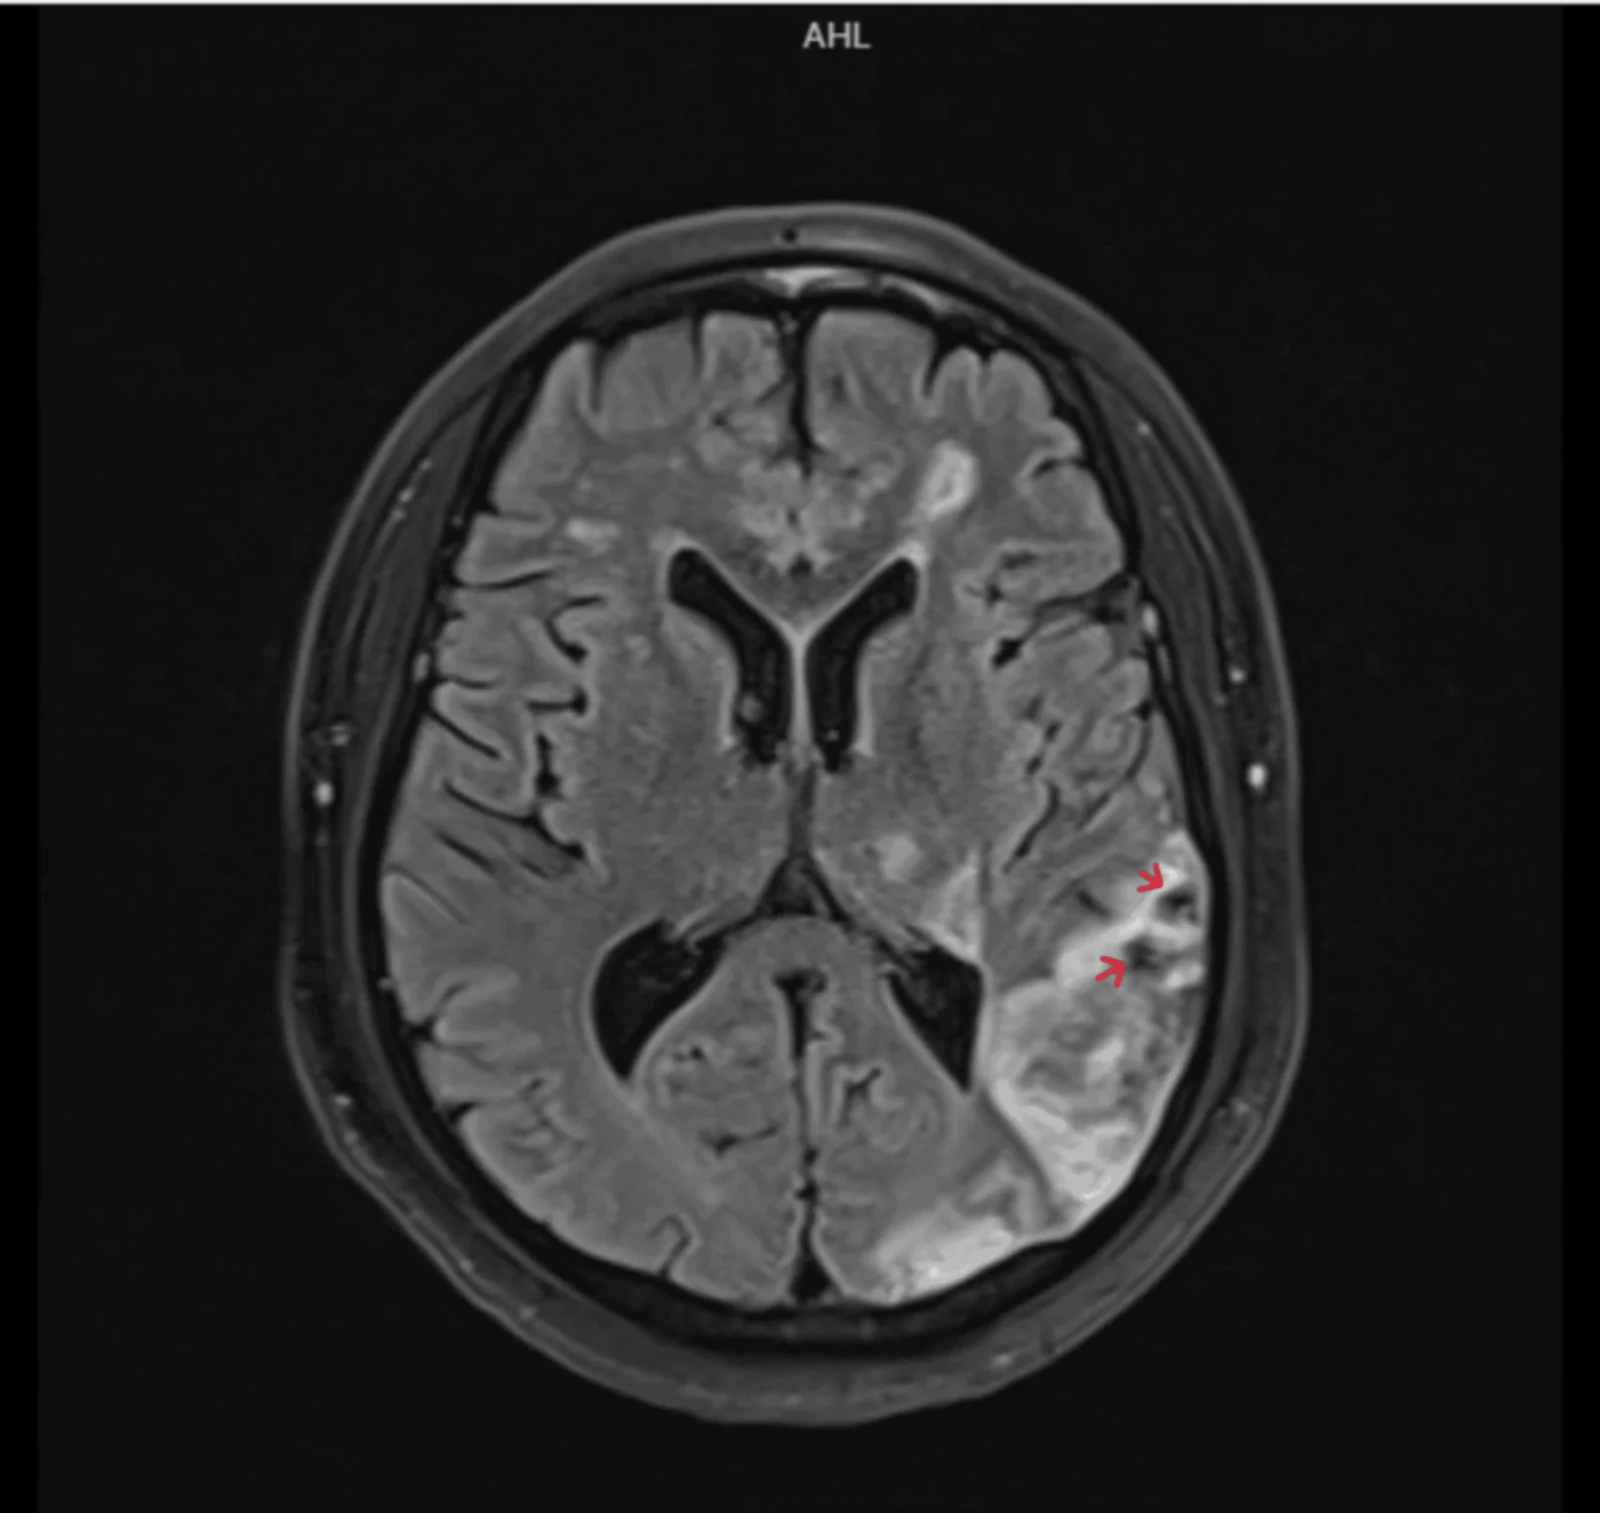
Post-thrombectomy MRI demonstrating acute left ICA territory infarction involving the hemisphere and thalamus, with evidence of focal hemorrhagic transformation in the parietal region (as indicated by red arrows). ICA: internal carotid artery
